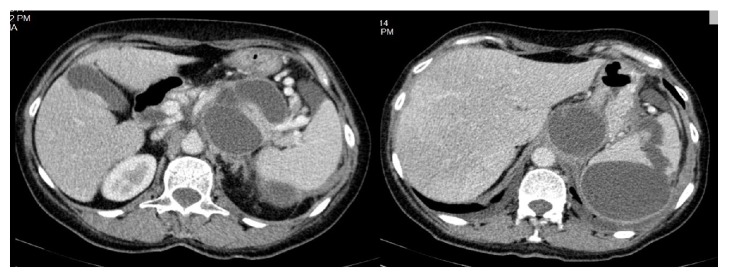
Preoperative CT scan showing 3 distinct cystic masses in the area of pancreas and spleen.
